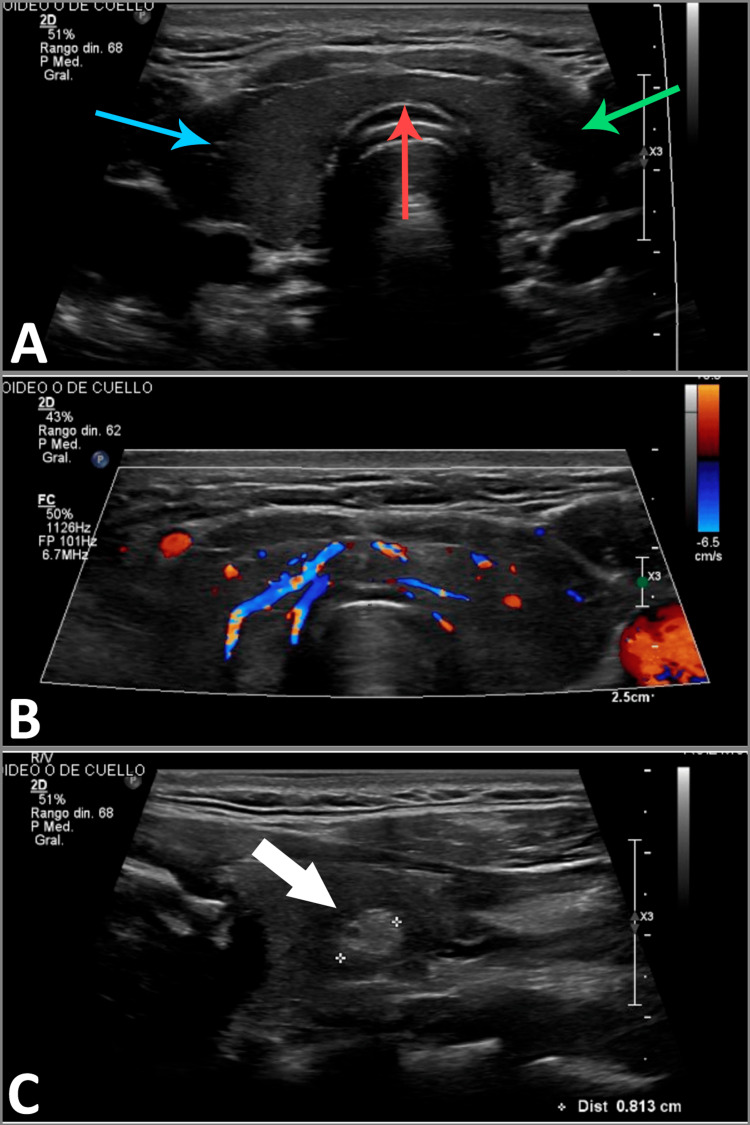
Thyroid gland ultrasound (A) shows thyroid in its normal position and morphology. The right lobe (blue arrow) has a volume of 4.8 mL, the left lobe (green arrow) has a volume of 8.3 mL, and the isthmus (red arrow) has a thickness of 5 mm. The parenchyma is homogeneous. In (B), preserved vascularity is observed with color Doppler. In (C), a hyperechoic image in the left lobe, oval-shaped, circumscribed, avascular, with a diameter of 5x6 mm is observed (Tirads 4a nodule). Tirads: Thyroid Imaging Reporting and Data System
